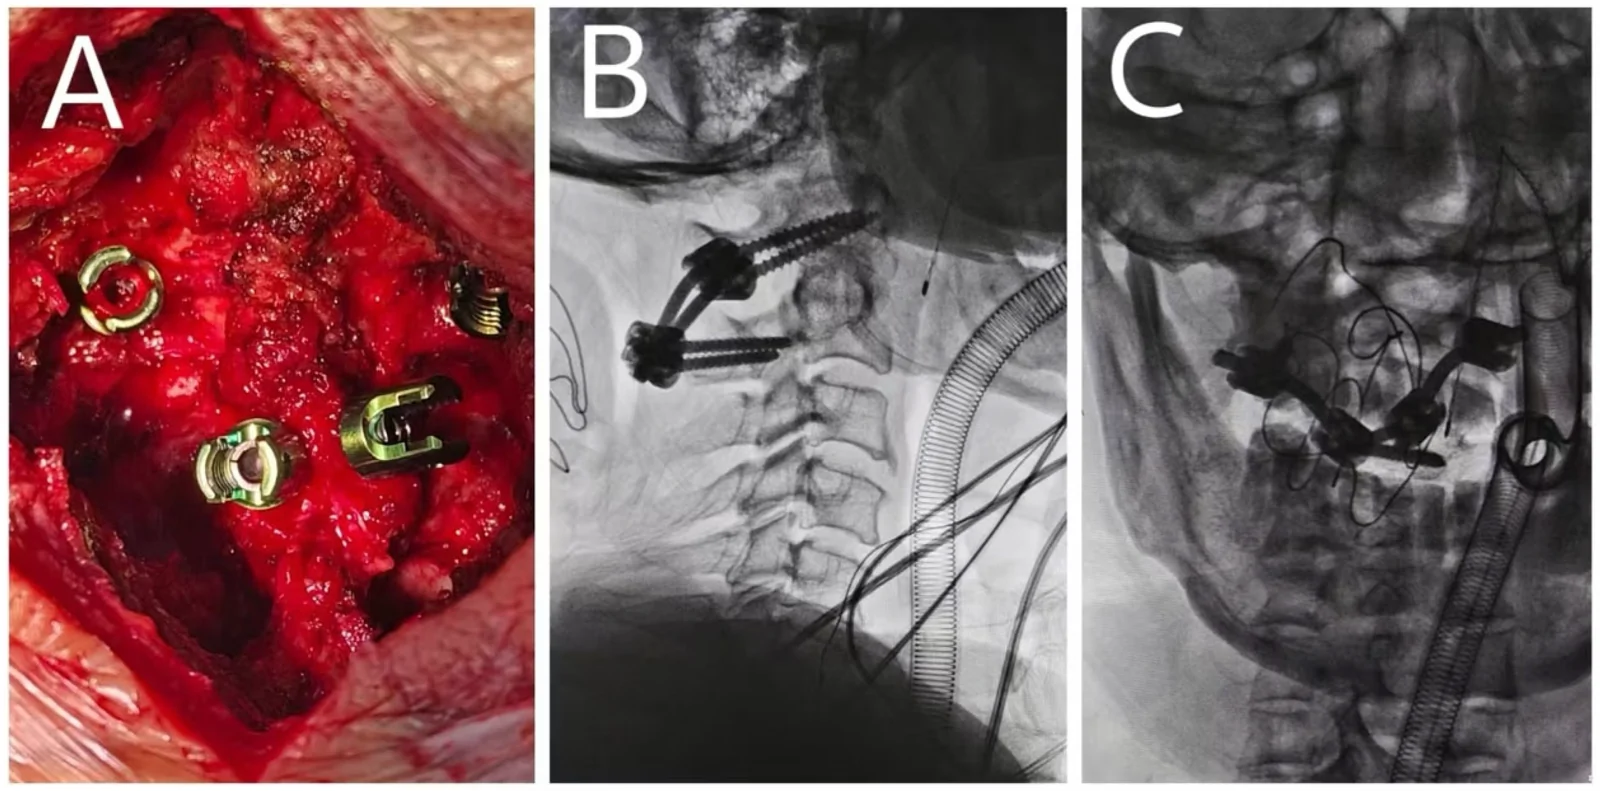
Intraoperative photograph and fluoroscopic images of C1 lateral mass screws combined with C2 laminar screw fixation: (A) intraoperative view showing implanted C1 lateral mass screws and C2 laminar screws; (B) lateral and (C) anteroposterior fluoroscopic radiographs of atlantoaxial screw placement.
